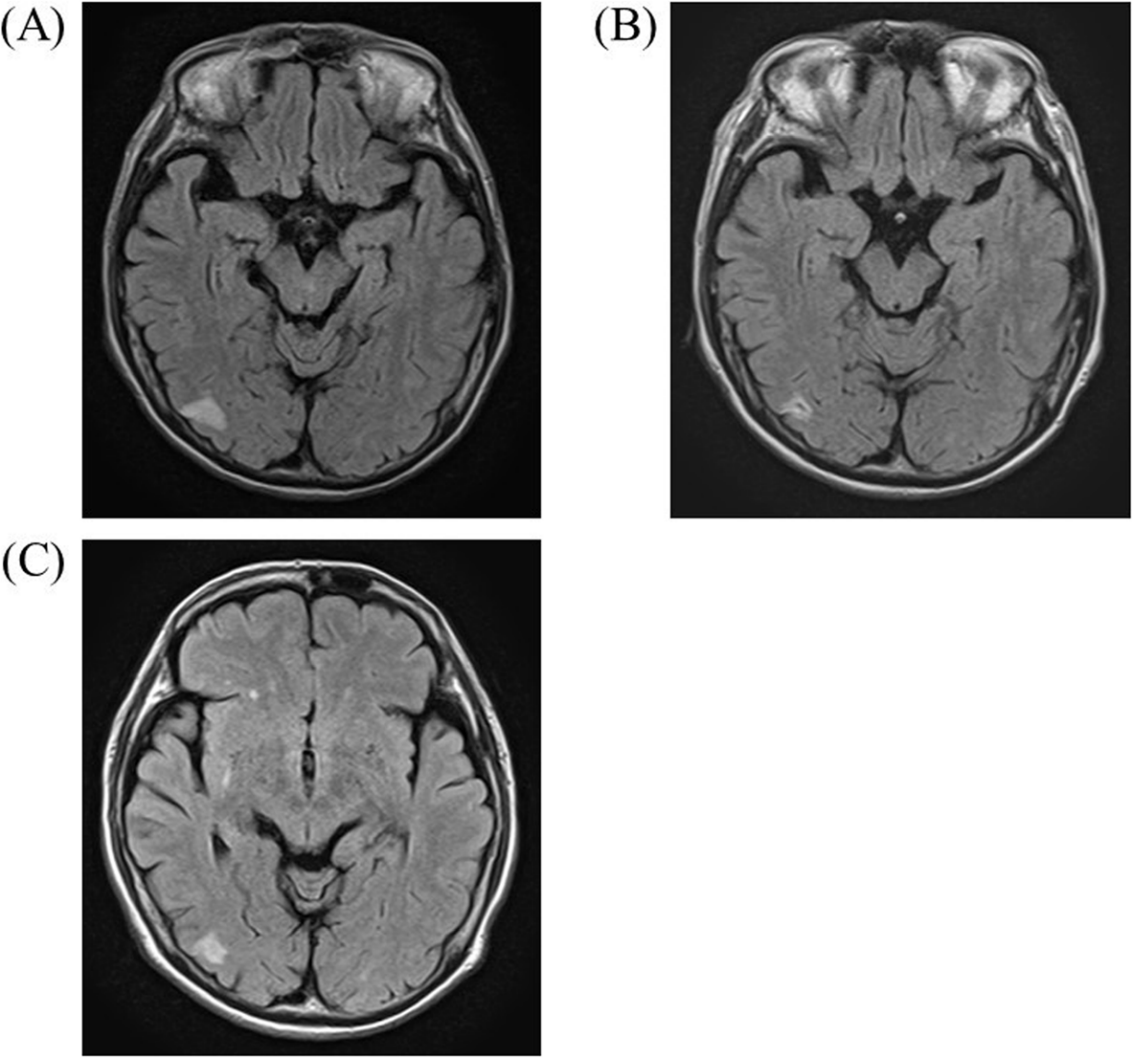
Brain magnetic resonance imaging. a: Before treatment, b: 4 months after the initial treatment, (c): 16 months after the initial treatment
